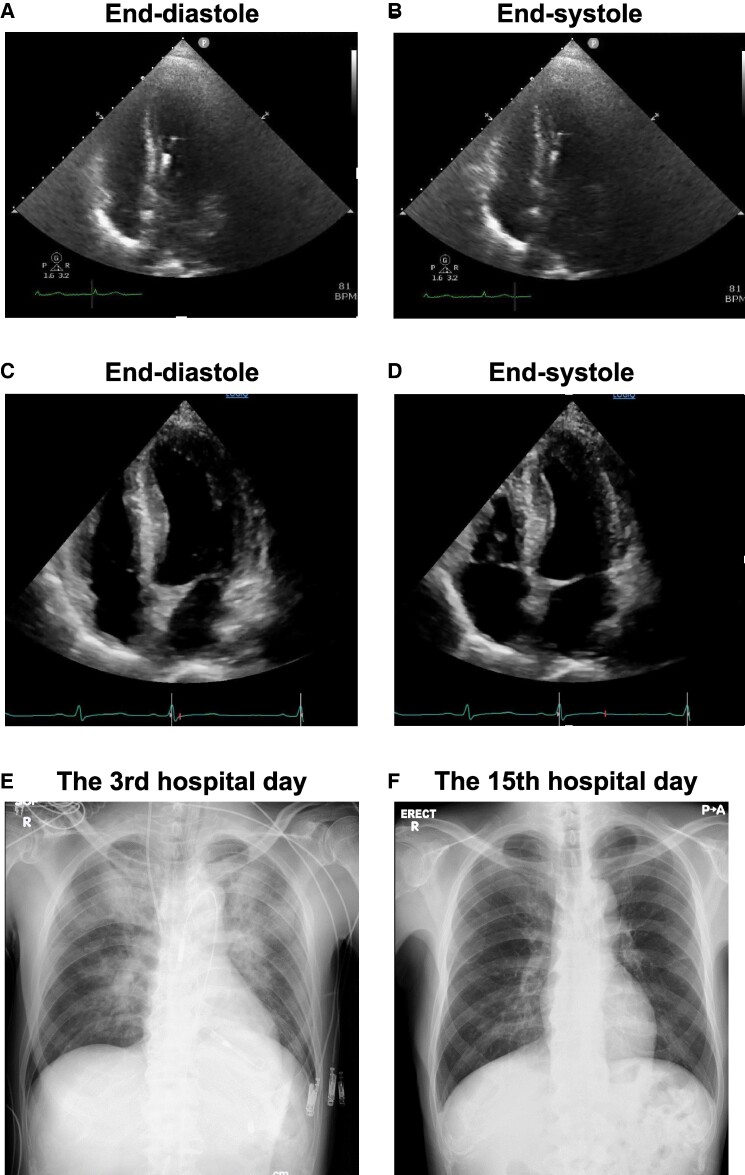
(*A* and *B*) Transthoracic echocardiography showing low left ventricular ejection fraction (38%) on hospital day 2. (*C* and *D*) Transthoracic echocardiography showing normal left ventricular ejection fraction (57%) on hospital day 11. (*E*) Chest radiography after Impella CP on hospital day 3. (*F*) Chest radiography showing improvement in cardiomegaly and pulmonary congestion on hospital day 15.
